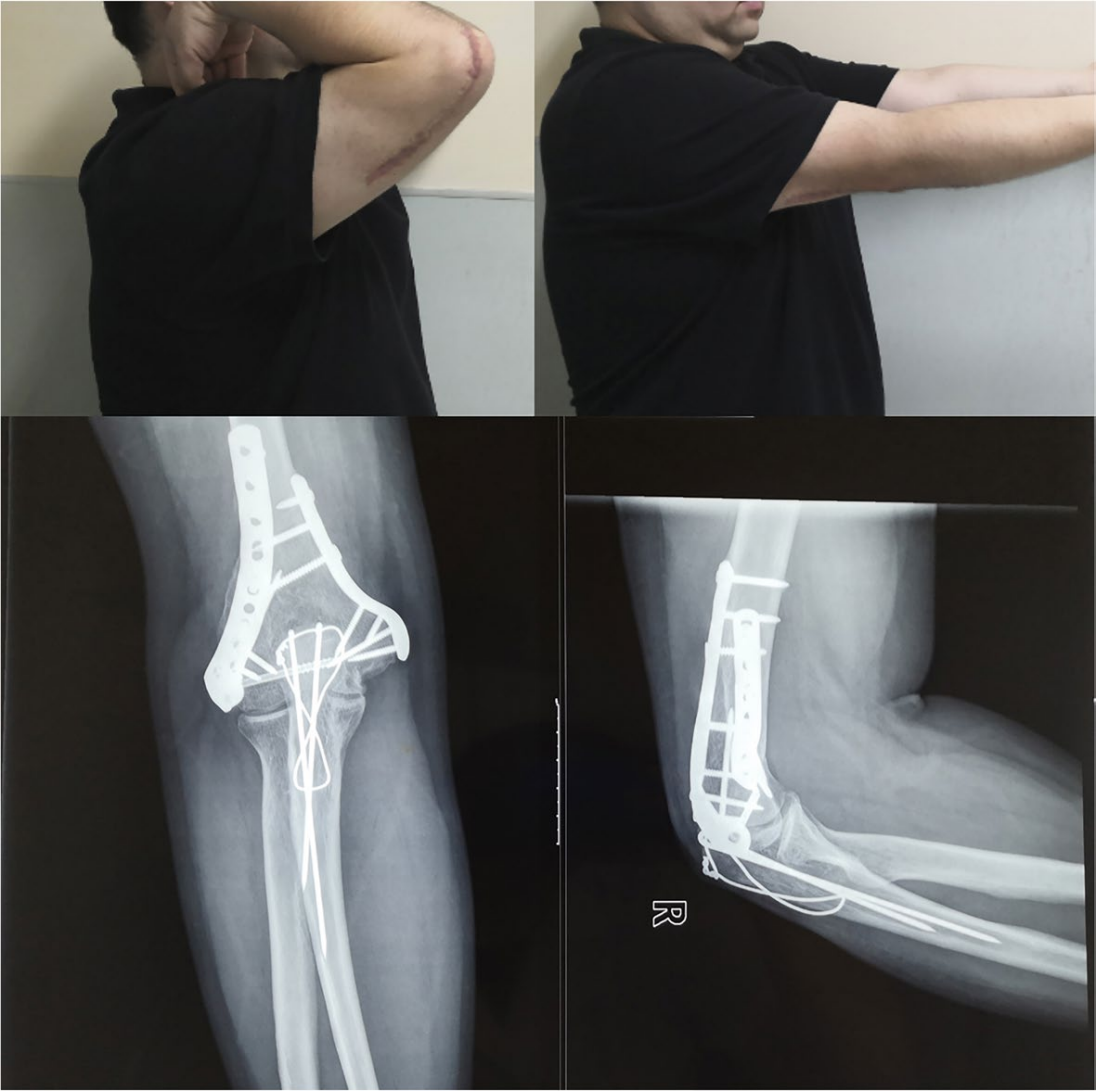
The elbow function and X-ray at the last follow-up
